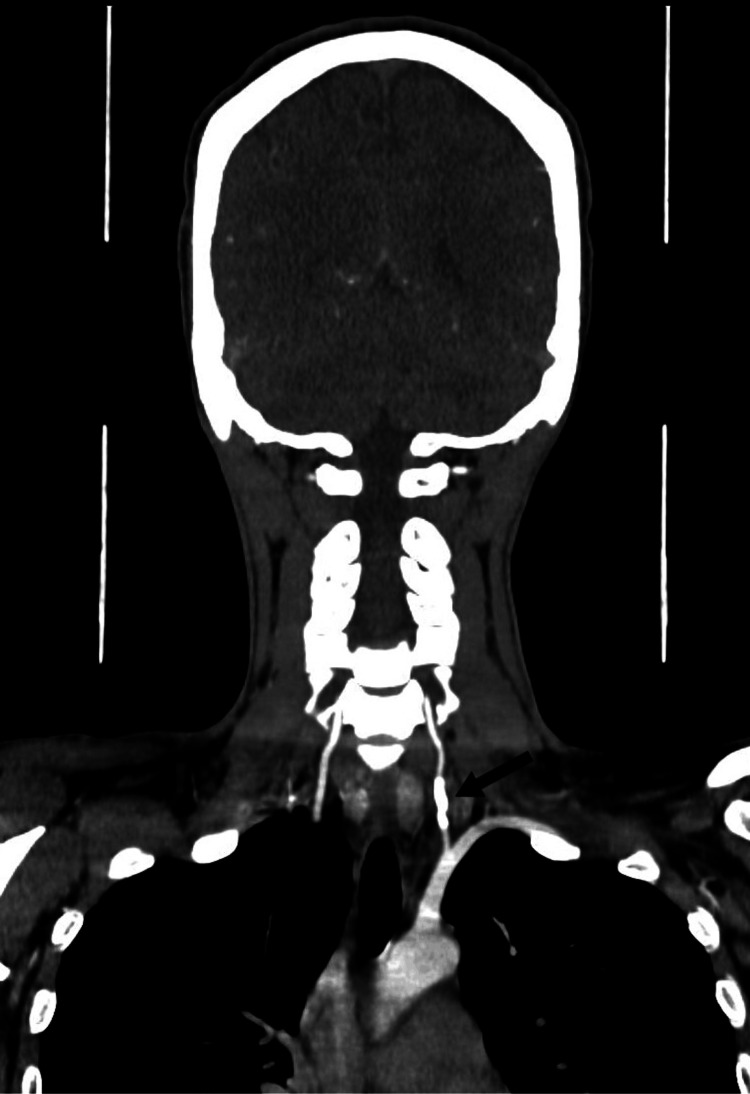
Computed tomography angiographic of the head, neck, and brain showing the patent stent in the left vertebral artery (arrow)
